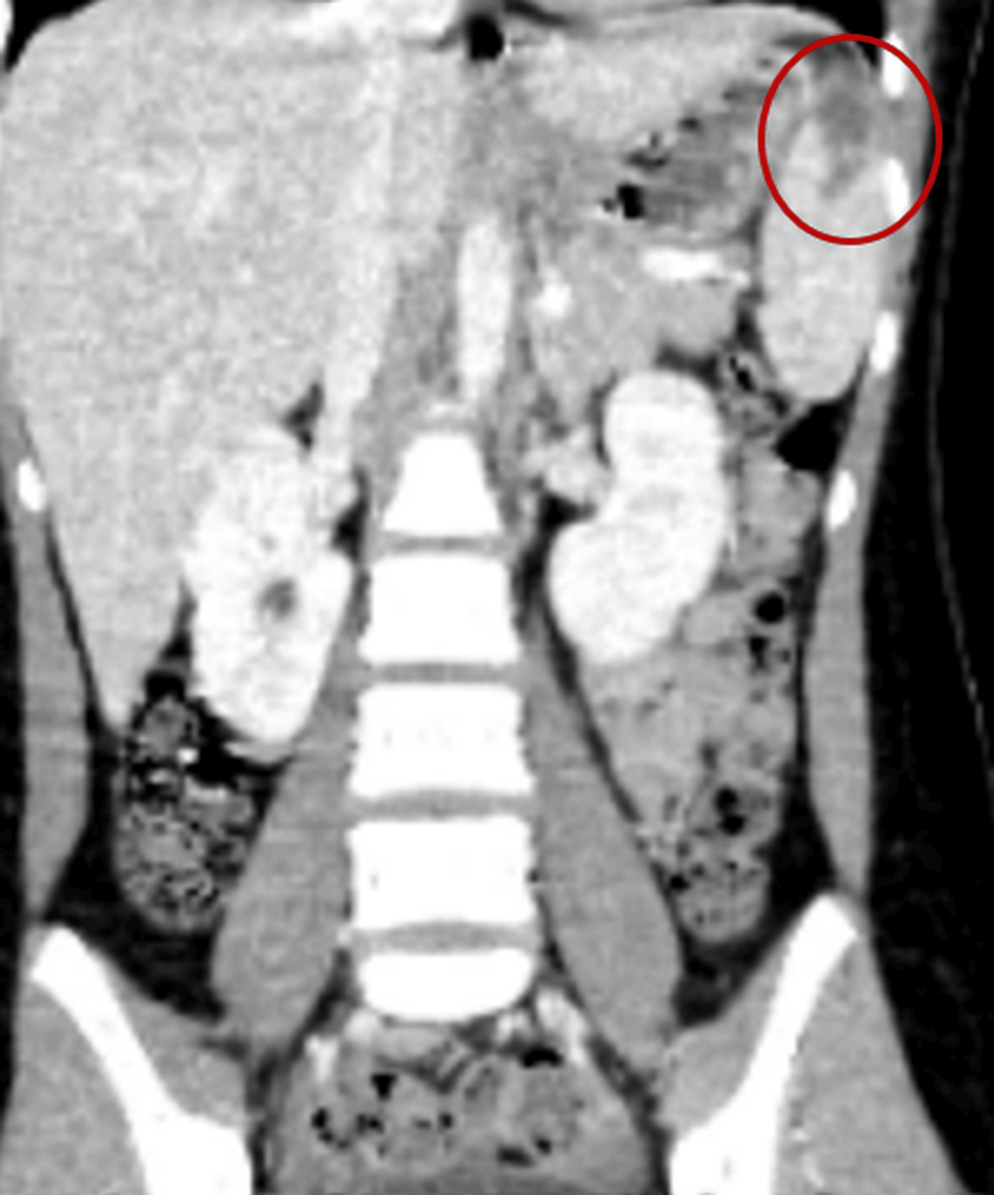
Coronal contrast-enhanced abdominal CT showing a well-defined hypodense lesion in the spleen (red circle), consistent with a splenic infarct
